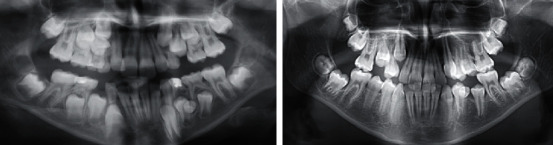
X-ray from the first clinical examination and 2 years later.
